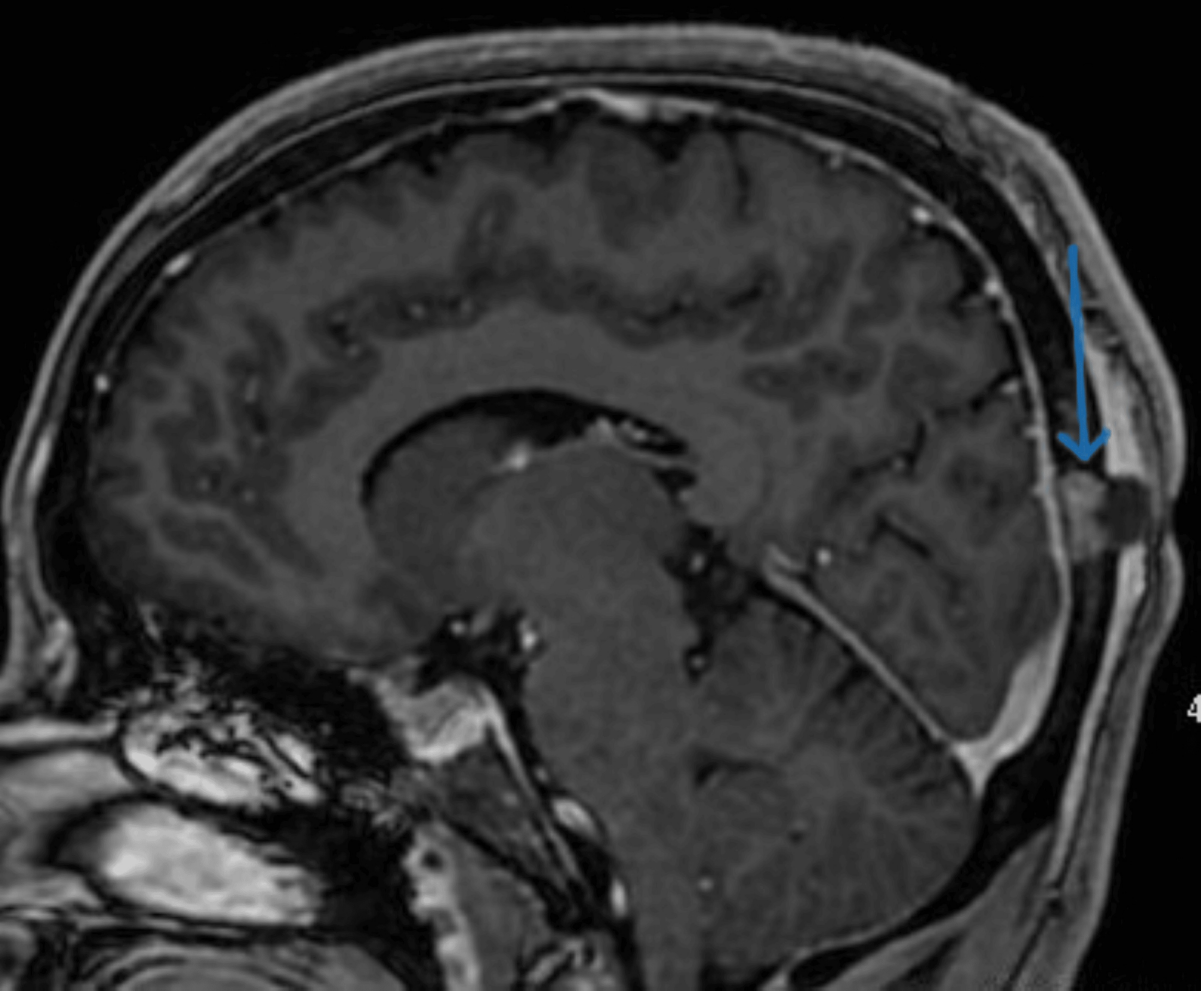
Enhanced sagittal T1 MRI of the brain demonstrates an occipital bone-enhancing lesion with minimal intracranial soft tissue component. No evidence of intraparenchymal lesion or invasion to the adjacent venous sinus is noted.
